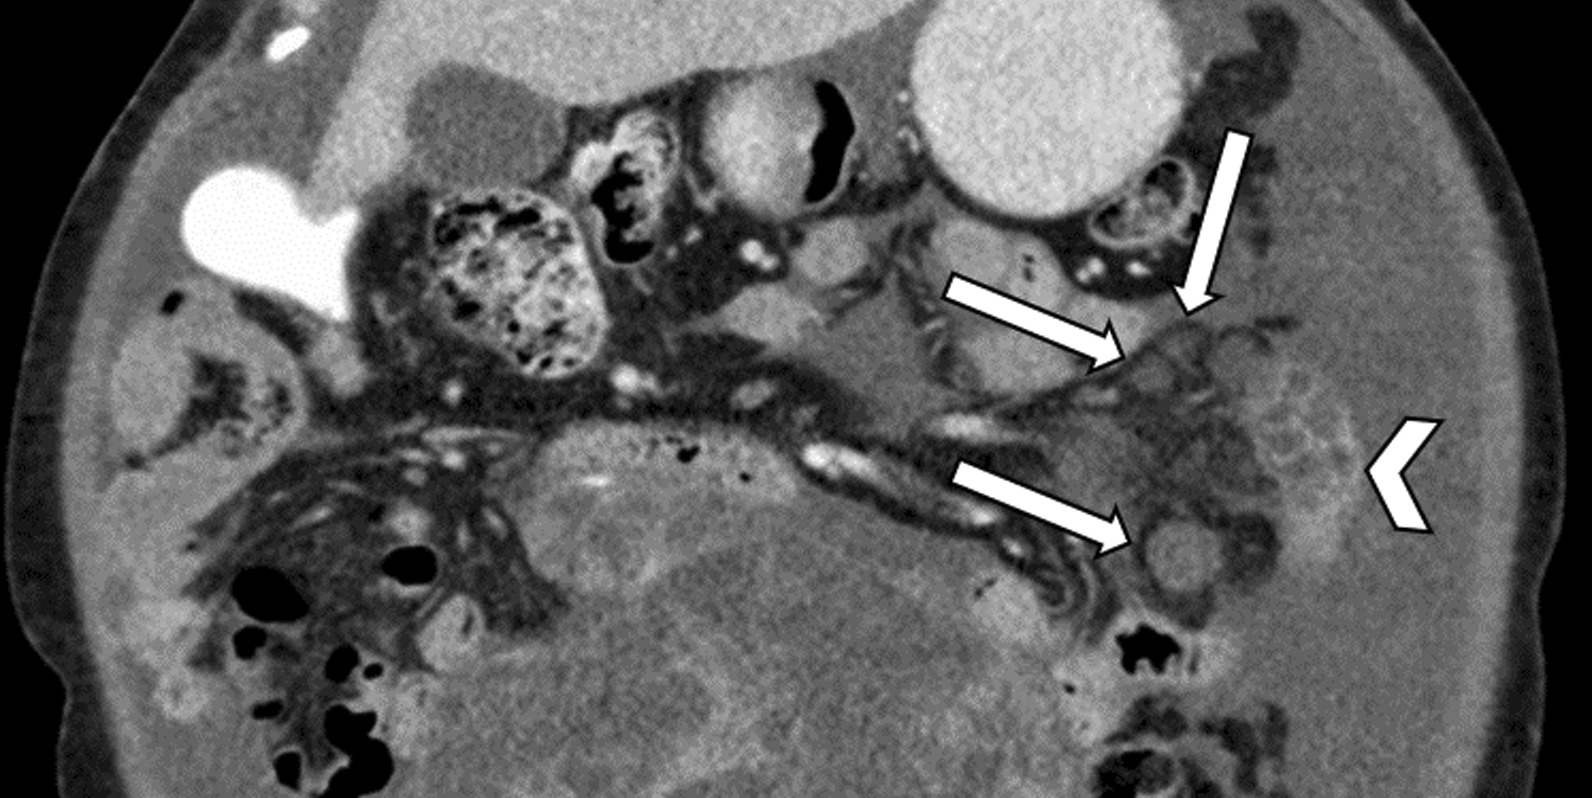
This portion of an abdominopelvic CT taken prior to the preexploratory laparotomy demonstrates hypodense spherical and ovoid locules (arrows) within the mesentery and adjacent to the small bowel (arrowhead)
